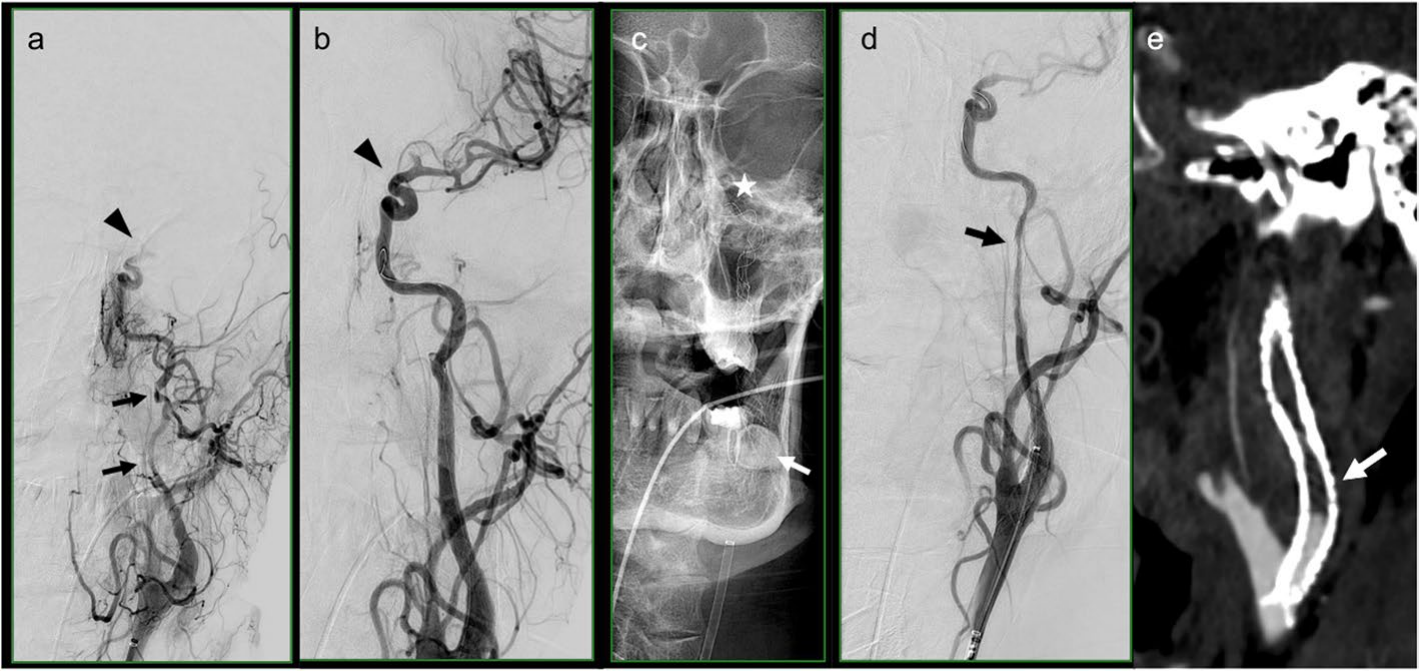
DSA image of a 56y old patient with cervical ICA dissection (arrows in a) and MCA occlusion (arrowhead in a). The MCA was recanalized (arrowhead in b) and the ICA reconstructed using three overlapping stents (c: arrow shows proximal end before placement of 3rd stent, asterisk distal end). Despite i.v. eptifibatide infusion and prior aspirin intake, in-stent thrombosis occurred at the end of the intervention (arrow in d). Control CTA 12 h post intervention showed occlusion of the stents (arrow in e). DSA: Digital subtraction angiography, ICA: Internal carotid artery, MCA: Middle cerebral artery, CTA: Computerized Tomography Angiography
